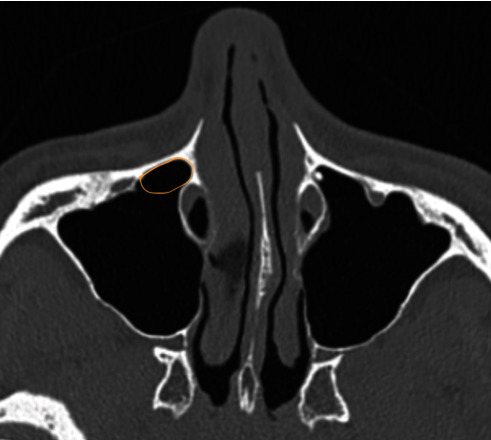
CT representation of the prelacrimal recess shown bounded by the anterior and medial walls of the maxillary sinus, nasolacrimal duct, and infraorbital nerve. The medial boundary defined as the bone between the pyriform aperture and nasolacrimal duct can be instrumented to provide surgical access to the anterior and inferior walls of the maxillary sinus.
